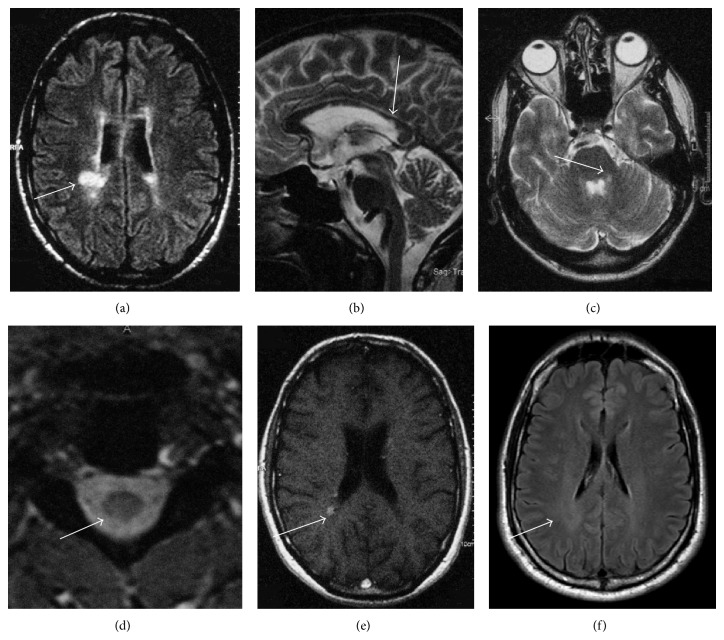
Brain and spinal cord MRI of the patient with CMTX and MS and brain MRI of his first cousin with CMTX and asymptomatic diffuse CNS involvement. (a) Axial FLAIR brain MRI of the patient demonstrating periventricular lesions characteristic of MS. (b) Sagittal T2 brain MRI of the patient revealing lesions in the corpus callosum characteristic of MS. (c) Axial T2 brain MRI of the patient demonstrating a lesion in the left middle cerebellar peduncle. (d) Axial T2 cervical cord MRI of the patient demonstrating a spinal cord lesion. (e) Axial T1 with IV contrast brain MRI of the patient showing periventricular gadolinium-enhancing lesions suggesting inflammation. (f) Axial FLAIR brain MRI of the patient's first cousin revealing diffuse white matter hyperintensity more prominent posteriorly.
